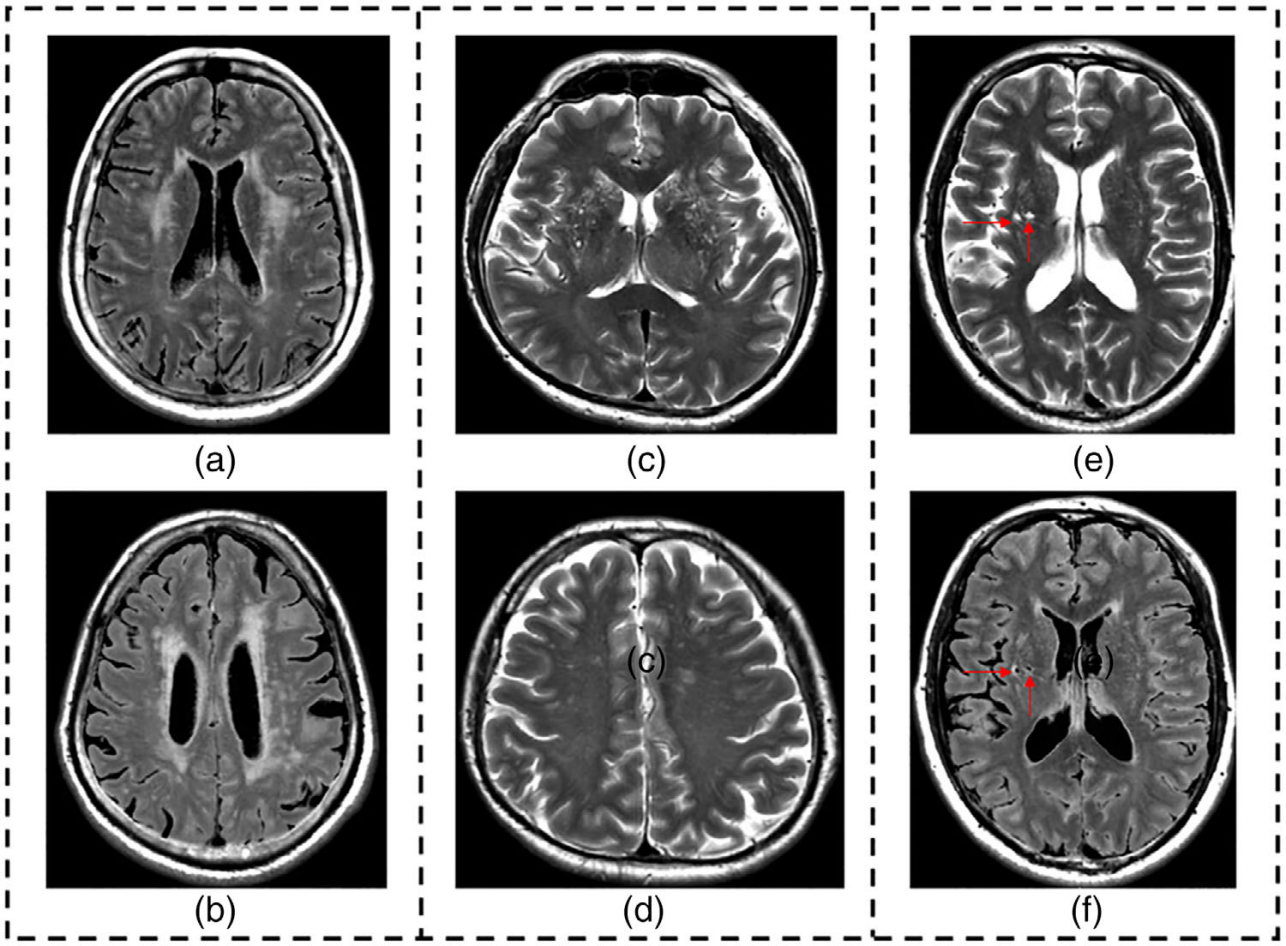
Imaging markers of cerebral small vessel disease. (a) Deep white matter hyperintensities; (b) periventricular white matter hyperintensities; (c) enlarged perivascular space in the basal ganglia; (d) enlarged perivascular space in the centrum semiovale; (e) lacunes on T2‐weighted magnetic resonance images (red arrows); (f) lacunes on fluid‐attenuated inversion recovery images (red arrows)
